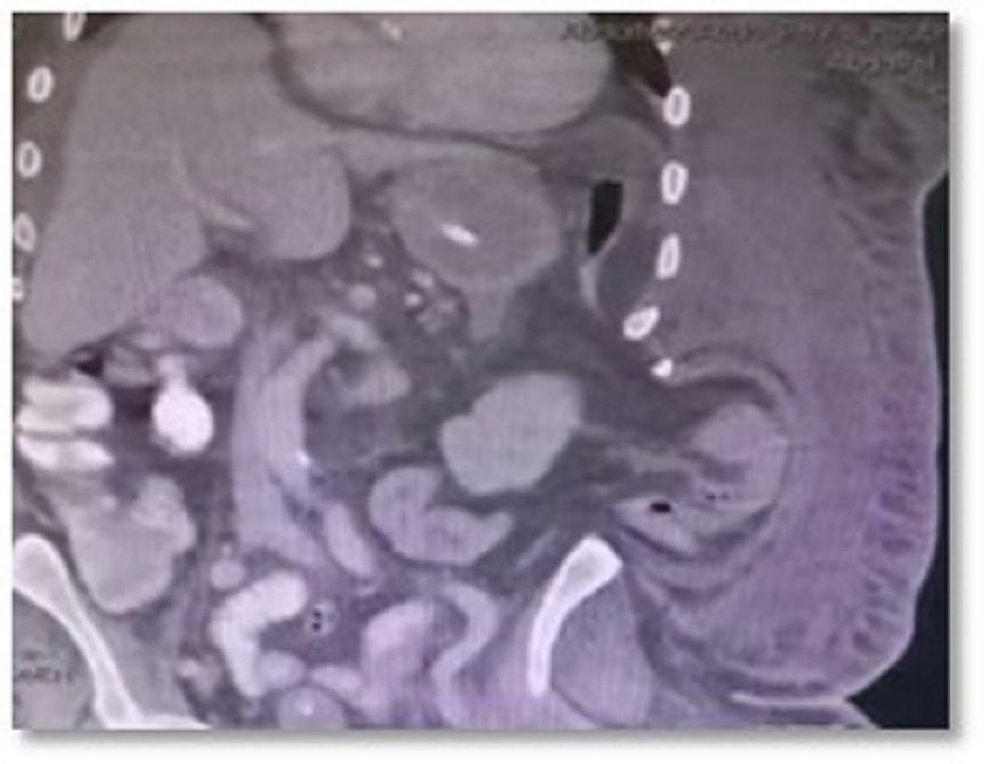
A CT scan of the abdomen and pelvis A CT scan of the abdomen and pelvis with oral contrast showing fluid accumulation in the left upper abdomen, related to the stomach and extending inferiorly. It measured about 5.7 x 8.2 x 9.8 cm in size. A fluid collection of 6.9 x 7.3 x 1.7 cm was also found superior to the left hepatic lobe. There was no CT evidence of intra-abdominal free air or contrast leak.
